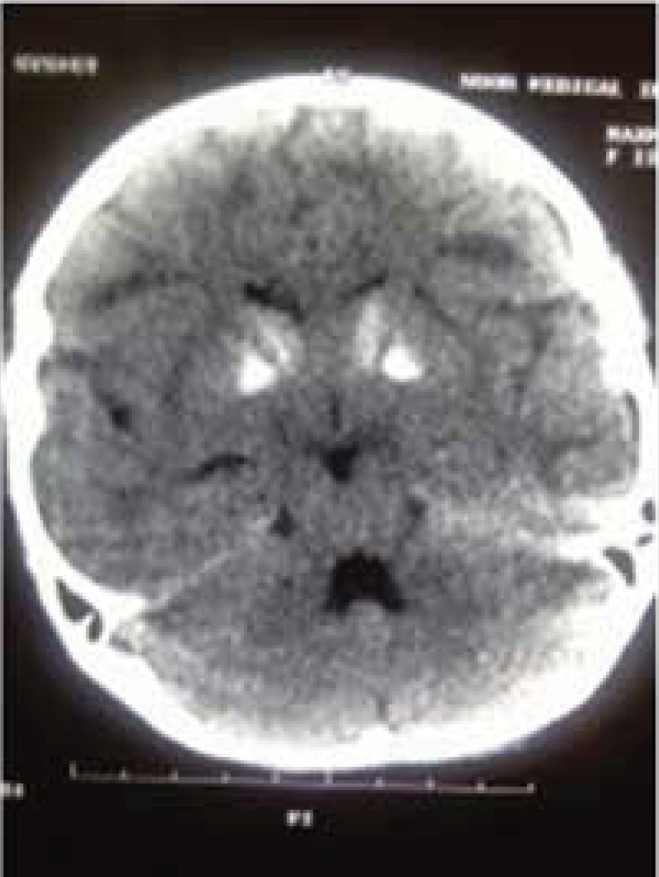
Brain CT scan shows bilateral calcification in basal ganglia, periventricular demyelination and mild dilatation of lateral ventricles
